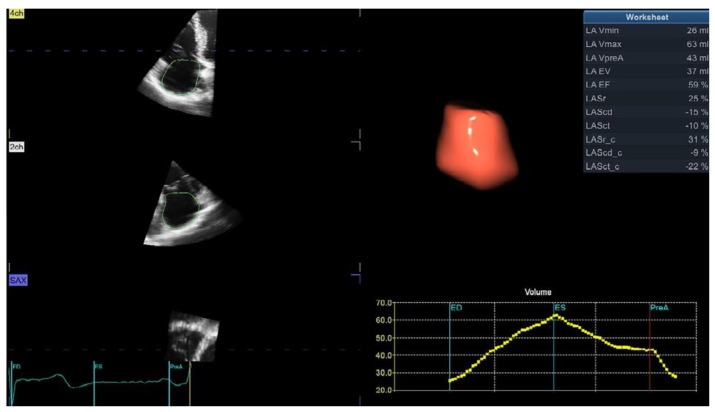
Three-dimensional echocardiographic volume- and strain-derived right atrial phasic analysis (software is dedicated for left atrial analysis and, therefore, all acronyms are devoted to the left atrium (LA) and should be replaced with the right atrium (RA) as the same software is used off-label for the RA). ED—end-diastole, ES—end-systole, LA EV—left atrial emptying volume, LA EF—left atrial emptying fraction, LASr—left atrial longitudinal strain during reservoir phase, LAScd—left atrial longitudinal strain during conduit phase, LASct—left atrial longitudinal strain during contraction phase, LASr_c—left atrial circumferential strain during reservoir phase, LAScd_c—left atrial circumferential strain during conduit phase, LASc_ct—left atrial circumferential strain during contraction phase, LA Vmax—maximum left atrial volume, LA Vmin—minimum left atrial volume, LA VpreA—left atrial volume before atrial contraction, pre-A—before atrial contraction.
